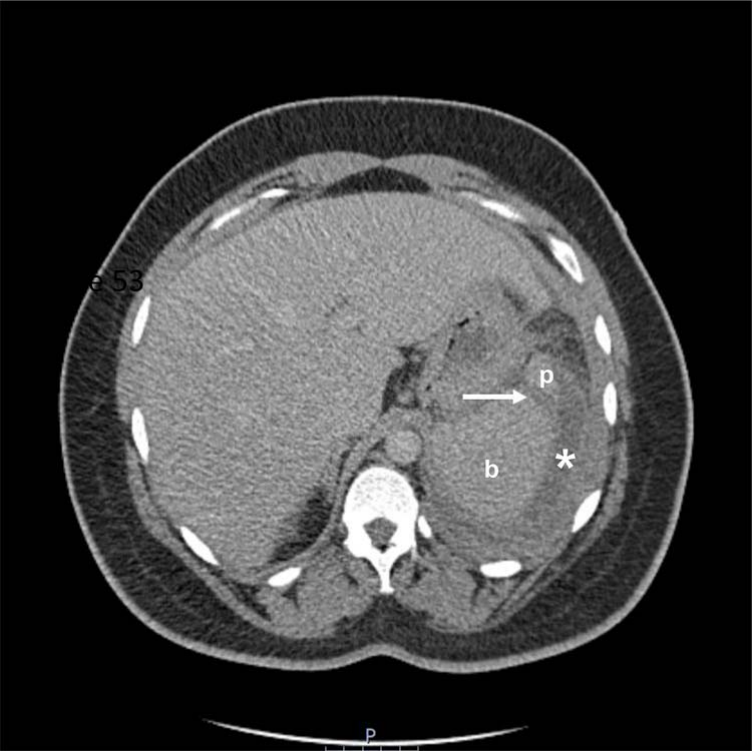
Two-mm axial section from a CT scan showing the perisplenic haematoma (*) and hypodensity (arrow) separating the upper anterior pole of the spleen (p) from the body (b). CT section number 54.
